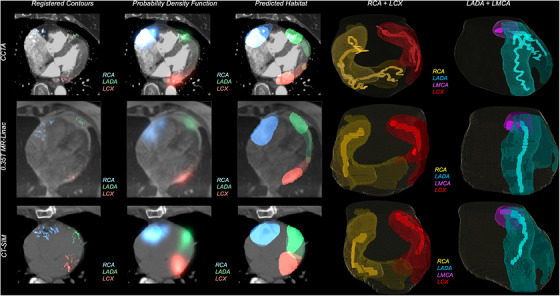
Registered contours, probability density functions, and predicted habitats (left) for example CCTA (top), 0.35T MR‐linac, (middle), and CT‐sim (bottom) cases. Volumetric renderings (right) of CAs overlaid with corresponding predicted habitats are shown for each case. Main‐branch habitats have brighter renderings while full‐branch habitats are represented by the larger, lightly shaded volumes shaded, larger volumes in the Predicted Habitats column in the middle and volumetric renderings for the RCA+LCX and LADA+LMCA. Acronyms defined in text.
